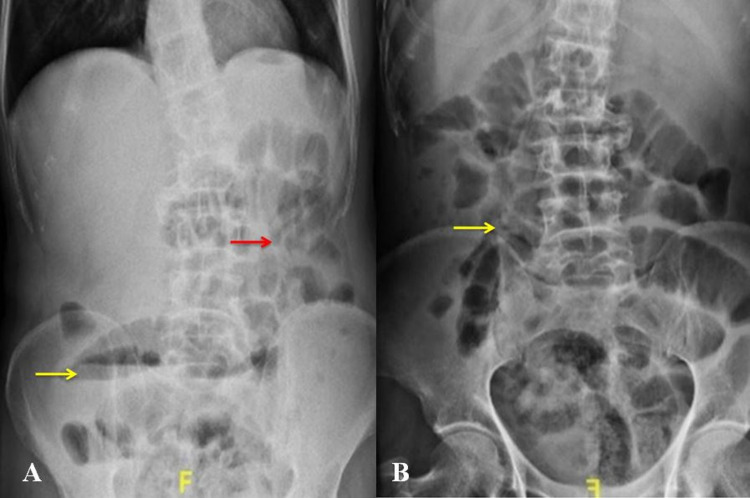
Plain radiograph of the abdomen showing (A) single air-fluid level (yellow arrow) with dilated small bowel loops (red arrow) and (B) few dilated bowel loops (arrow).
